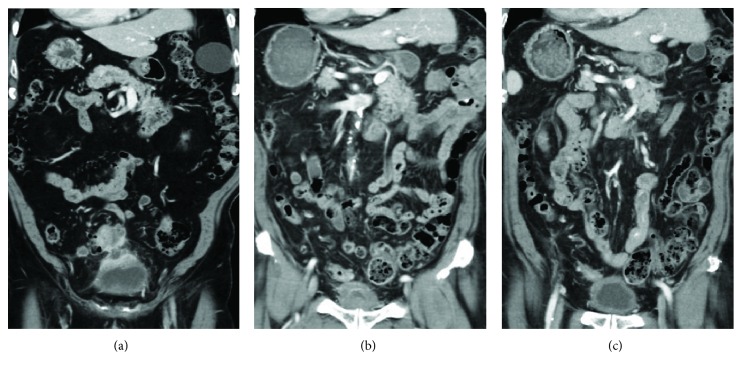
Before treatment, CT scan revealed a bulky mass in the sigmoid colon invading the bladder (a). After 6 courses of CAPOX (b). CT failed to detect the tumor after 13 courses of CAPOX plus bevacizumab (c).
